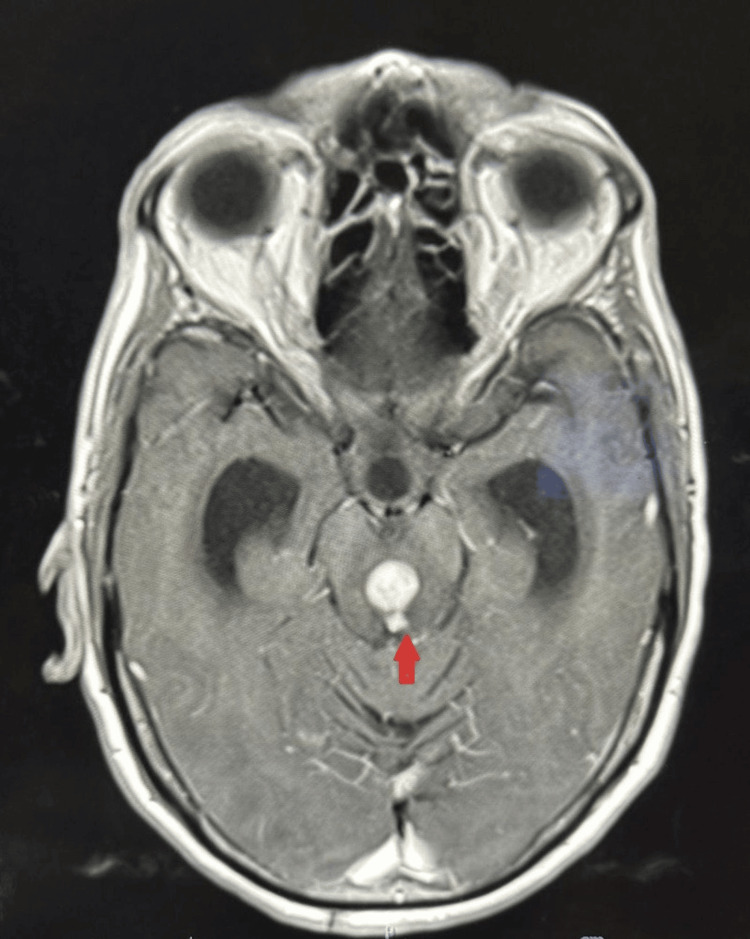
FLAIR: central hypointense lesion with surrounding hyperintensity suggestive of midbrain tuberculoma (red arrow) FLAIR: fluid-attenuated inversion recovery
